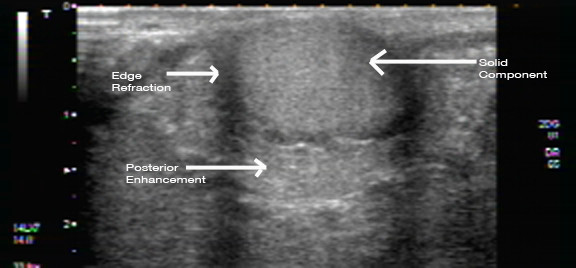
Ultrasound image of a palpable lump behind the nipple of the pregnant woman in Figure 12. The mass also contained an internal hyperechoic solid component with edge refraction and through transmission of sound.
